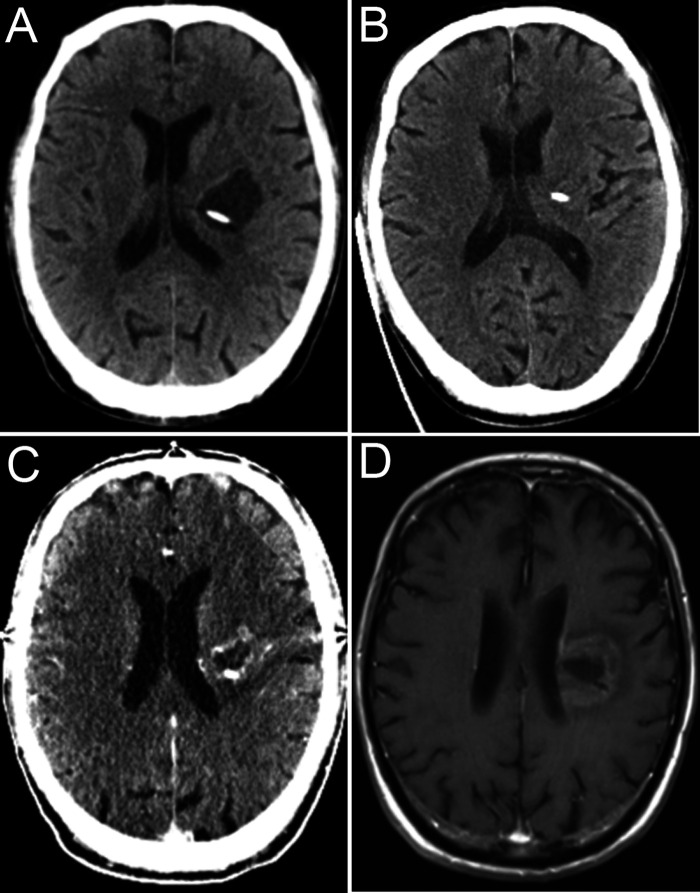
CT image showing fluid reaccumulation (A). CT image immediately after aspiration via the OR (B), CT image after first radiosurgery (C), and final imaging follow-up (D).
